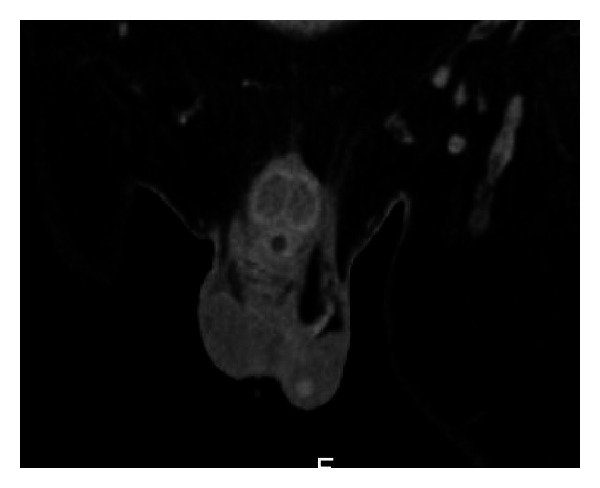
CT scan showing enhancing nodule in the left testicle consistent with malignancy.
